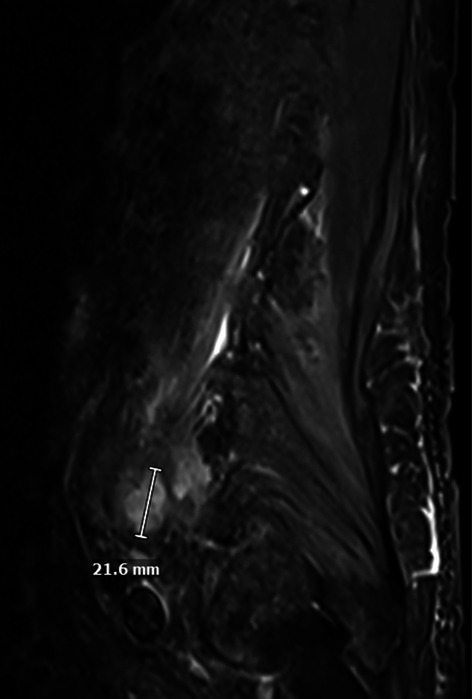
Sagittal magnetic resonance imaging view of left psoas abscess measuring 21.6 mm.
